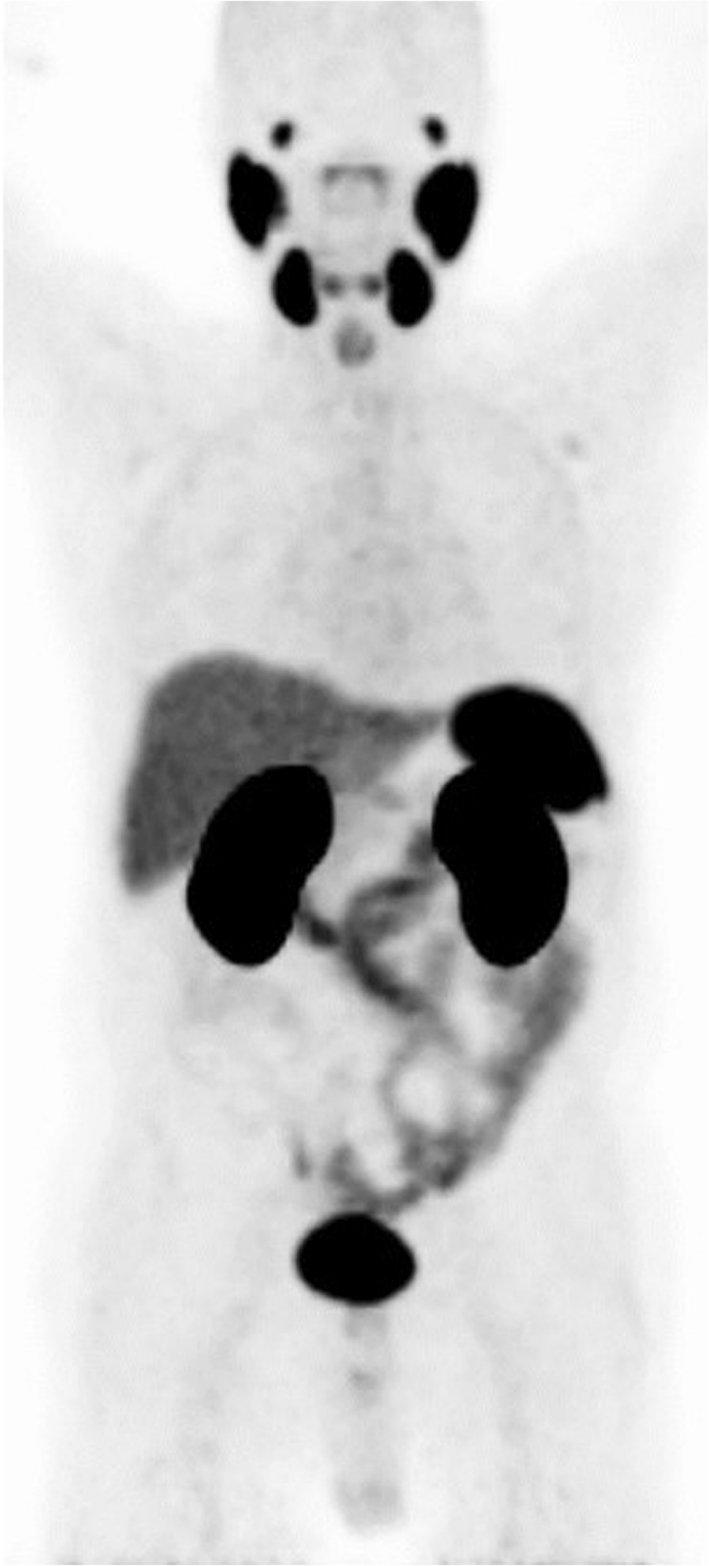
Maximum intensity protection (MIP) images of a patient with physiological distribution of ^68^Ga-PSMA ligand. Accumulation is seen in lacrimal and salivary glands, nasal mucosa, liver, spleen, bowel, kidneys and bladderFor ^68^Ga-PSMA ligand PET interpretation, first all PET positive lesions suspicious for PC are noted. In PET any focal uptake of ^68^Ga-PSMA ligand higher than the surrounding background and not associated with physiological uptake has to be considered suspicious for malignancy and judged and described below. As ^68^Ga-PSMA ligands are excreted via the kidneys and are highly accumulated in the urinary bladder, small local recurrences might be missed. Therefore it is especially important to evaluate PET images in axial as well as coronal and sagittal planes and to change the SUV-threshold to judge the PSMA-ligand uptake in soft-tissue structures near the urinary bladder. Examples of PC patients undergoing ^68^Ga-PSMA ligand PET/CT examination for primary staging and restaging are presented in Figs. 2 and 3.
